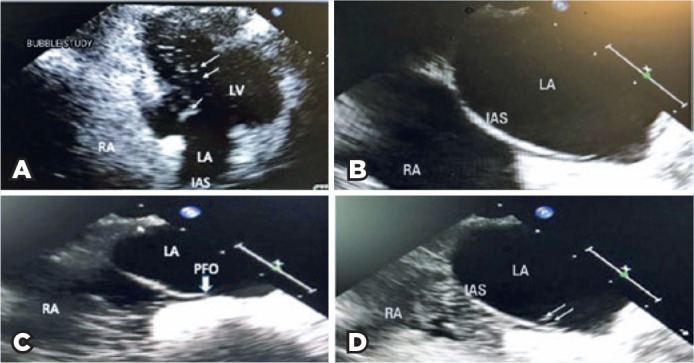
Echocardiographic images of a young patient with right central retinal
